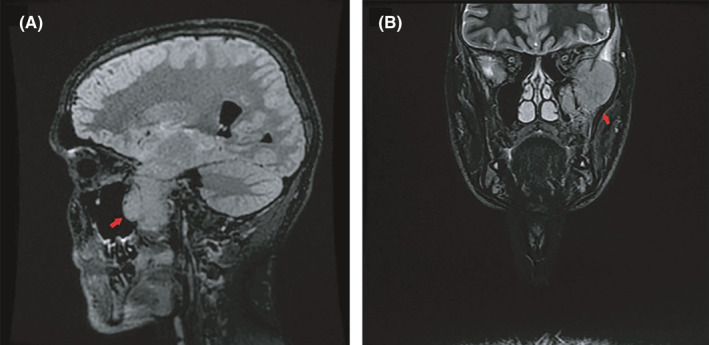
Sagittal (A) and coronal (B) MRI images demonstrating lesion in left temporal lobe eroding through base of skull with extracranial extension in the infratemporal fossa. Lesion extends up to pterygoid plates and posterior superior nasopharynx on the left side
